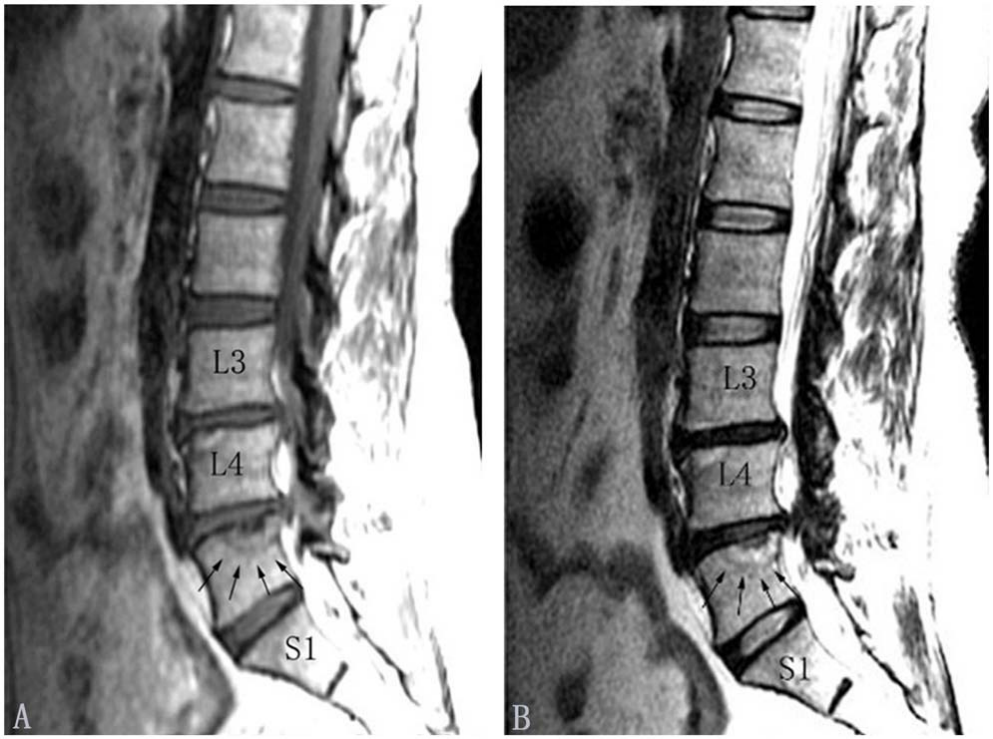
Modic type I changes in the lumbar spine (indicated by arrows). Modic type I changes appear hypointense on T1-weighted images (A) and hyperintense on T2-weighted images (B).
